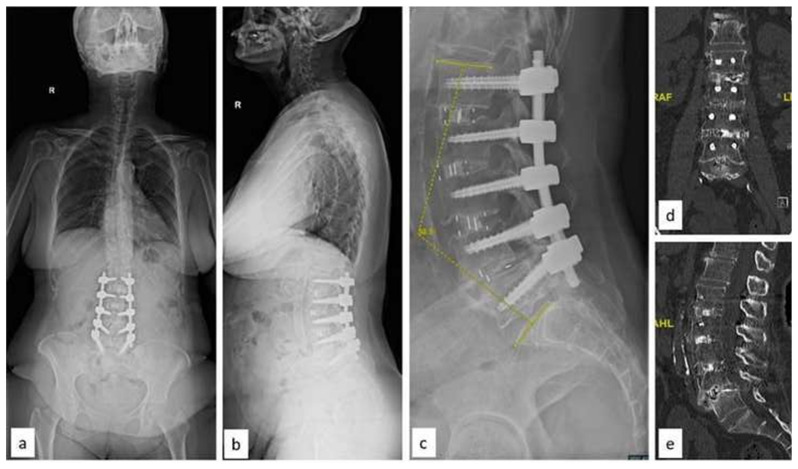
(a–b) Whole Spine AP and lateral radiographs following stage II: L4–L5 MIS TLIF with posterior pedicle screw fixation (c) Lateral lumbar radiograph showing correction of lumbar lordosis (L1–S1: 58.5°) (d–e) Four year follow-up CT scan showing solid fusion from L1–L5.
